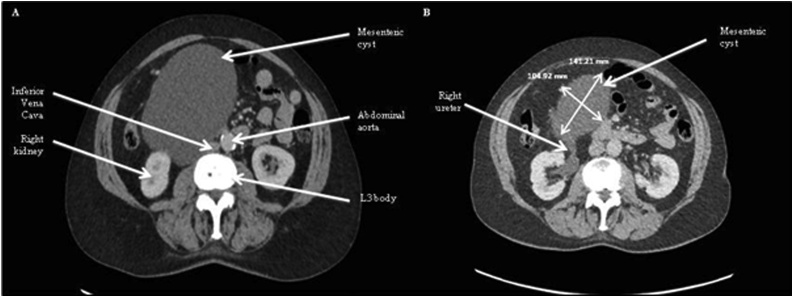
CT Abdomen and Pelvis demonstrating the anatomical relations of the mesenteric cyst (A). Note the relationship between the mesenteric cyst and the right ureter (B). Measurements of the cyst can also be found (B) although not to scale.
